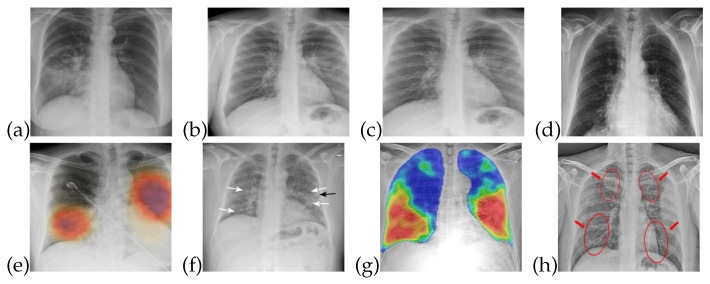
X-ray images for healthy and COVID-19 infected patients: (a–d) Normal patients X-ray (e–h) COVID-19 infected patients X-ray.
